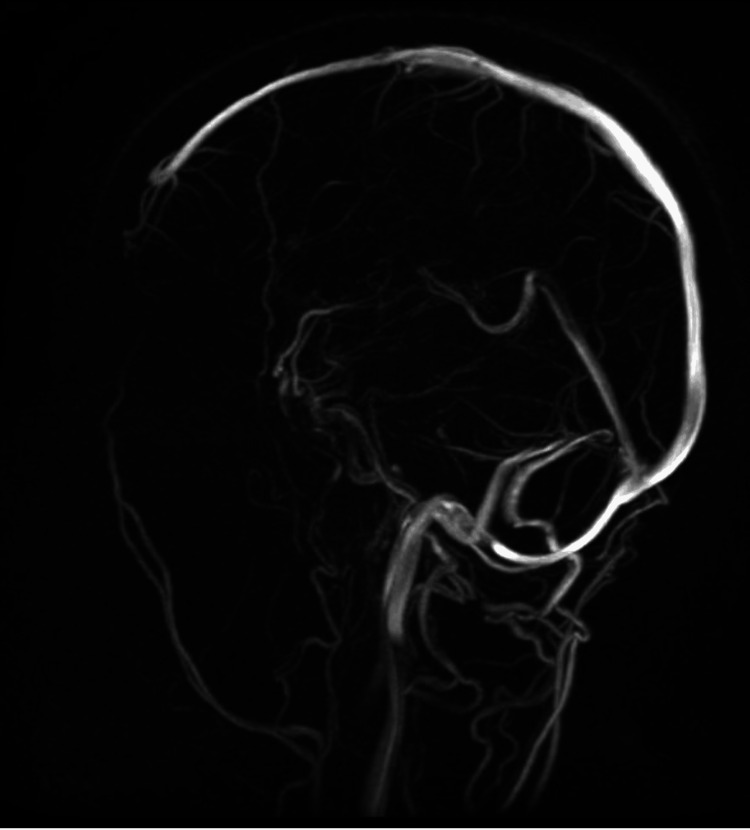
A magnetic resonance venography (sagittal view, T2-weighted) shows stenosis in the left transverse sinus
